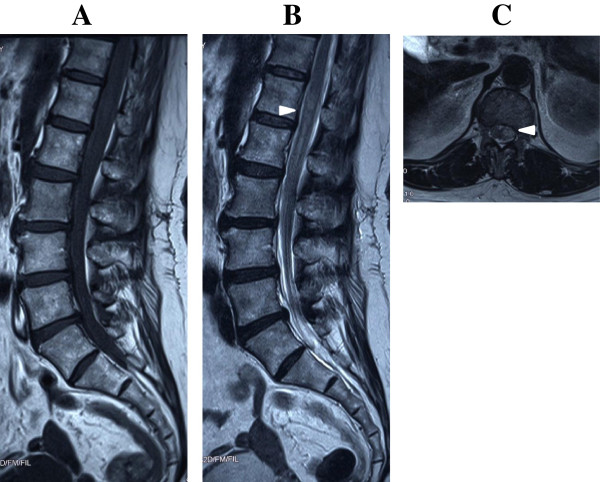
Initial magnetic resonance imaging. A sagittal T1-weighted image shows diffuse isosignal intensity in the subarachnoid space. No normal-appearing thecal sac or nerve roots are observed (A). Sagittal T2-weighted (B) and axial T2-weighted (C) images show a high signal intensity from L1 to L2 (arrowheads).
